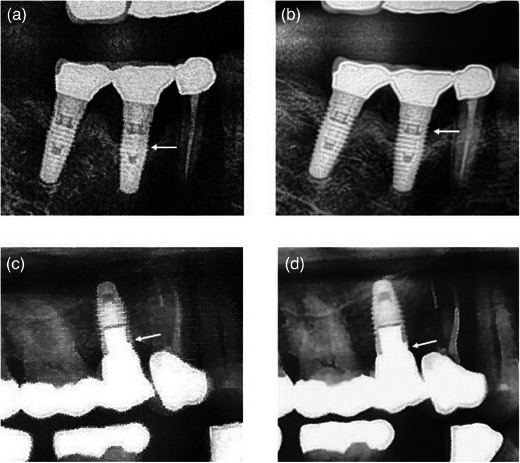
Periapical radiographs of implants at baseline (a), (c) and at follow up after treatment with hydrogel (b), (d). (a) Pretreatment bone attachment level of implant in the region of #46 with exposure of five implant threads. (b) Posttreatment radiographic bone level of implant in the region of #46 with exposure of three implant threads. (c) Pretreatment bone attachment level of implant in the region of #15 with exposure of three implant threads. (d) Posttreatment radiographic bone level of implant in the region of #15 with no exposure of implant threads.
